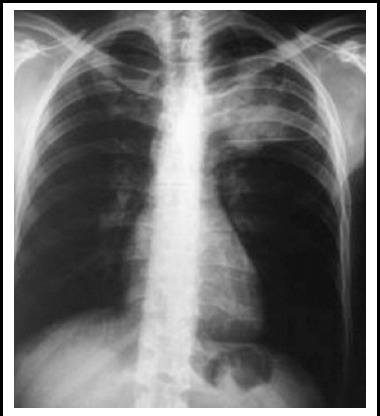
Chest x-ray shows soft tissue density in left upper lobe with a linear metallic foreign body along its inferior aspect.
